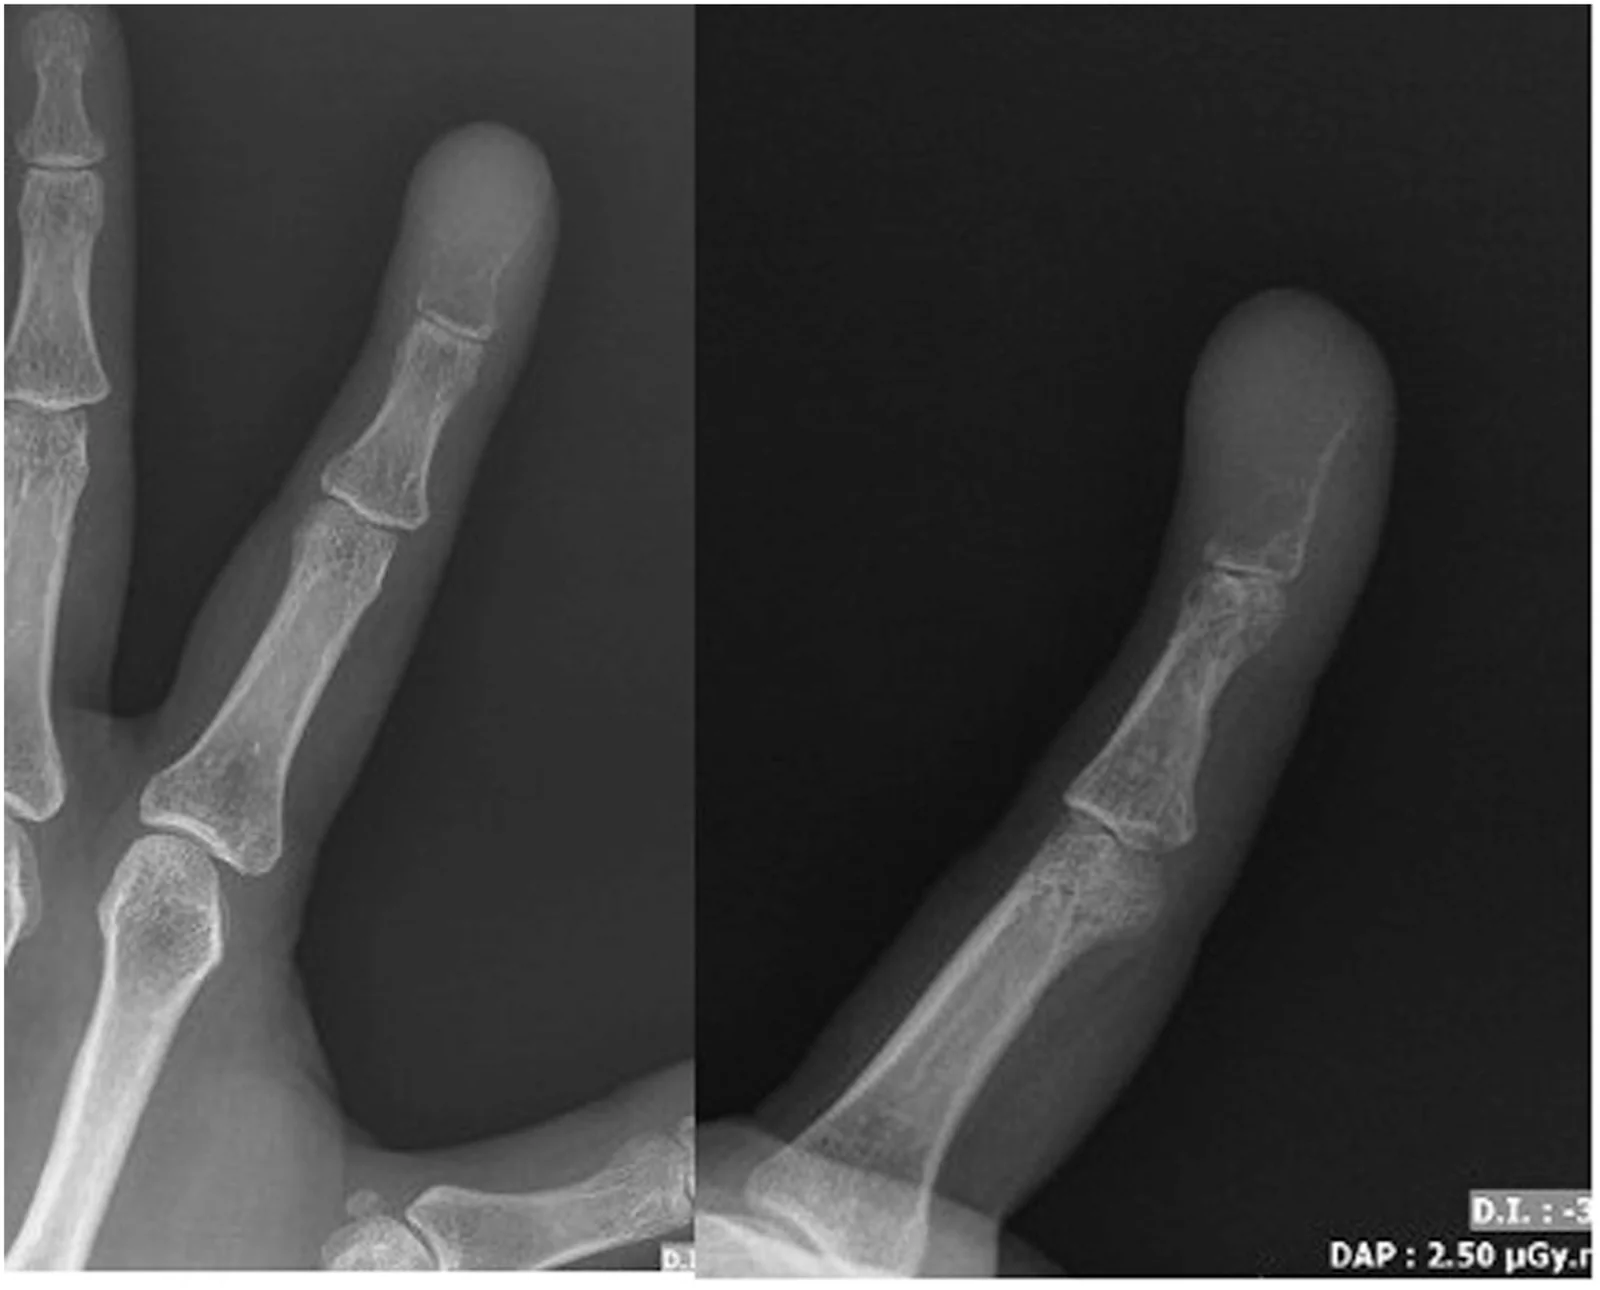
Plain x-ray. Demonstrating an expansile lytic lesion of the distal phalanx with dorsal cortex destruction.
